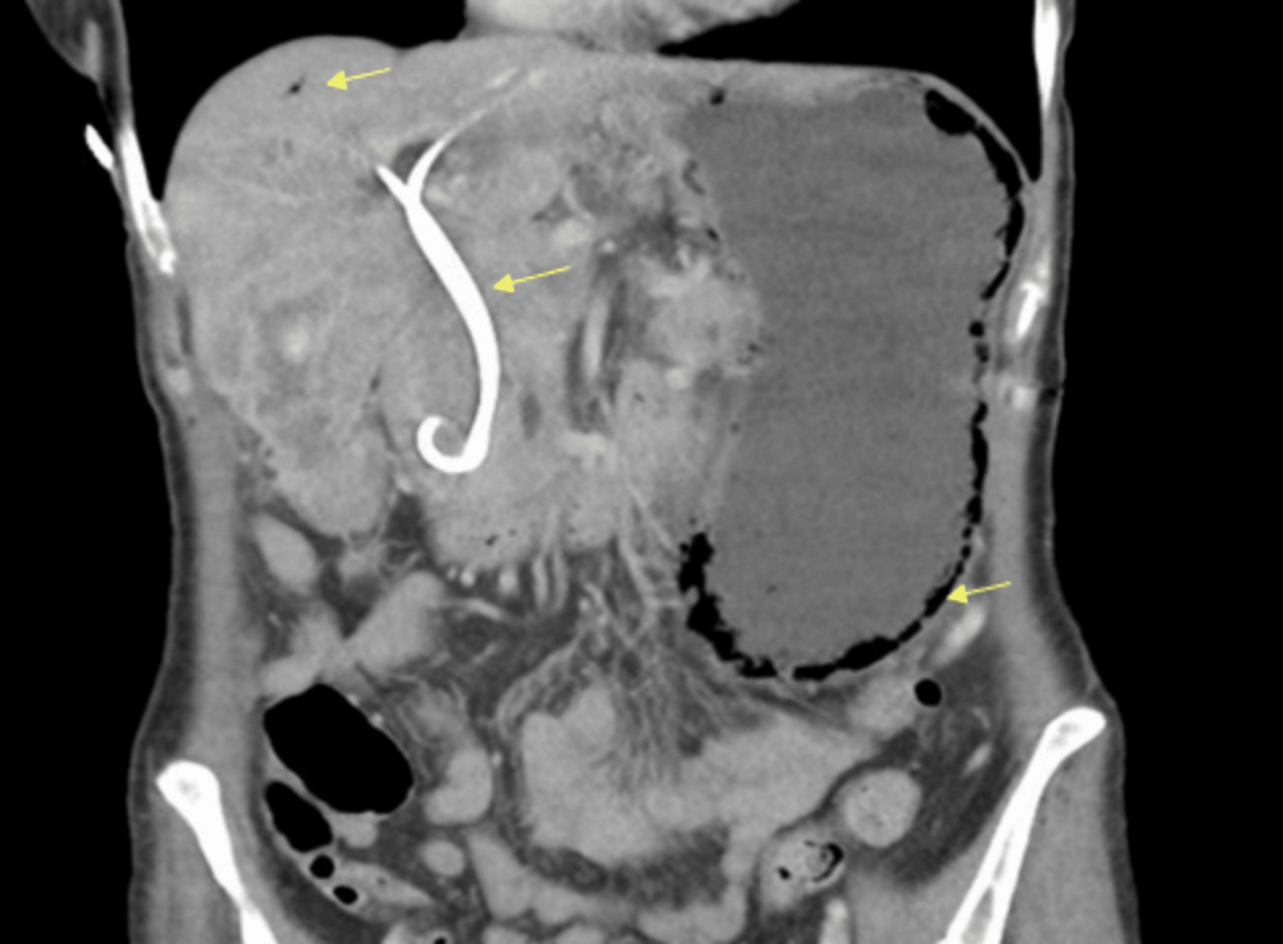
CT AP demonstrating significant gastric pneumatosis, a biliary stent and some portal venous gas in the setting of the known gallbladder small cell carcinoma
